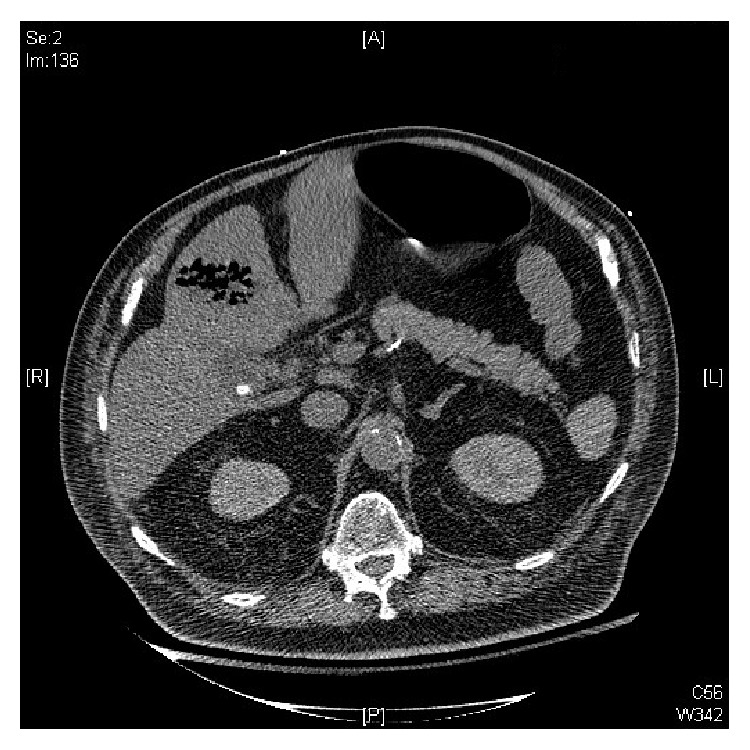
CT abdomen showing gas in left liver lobe.
